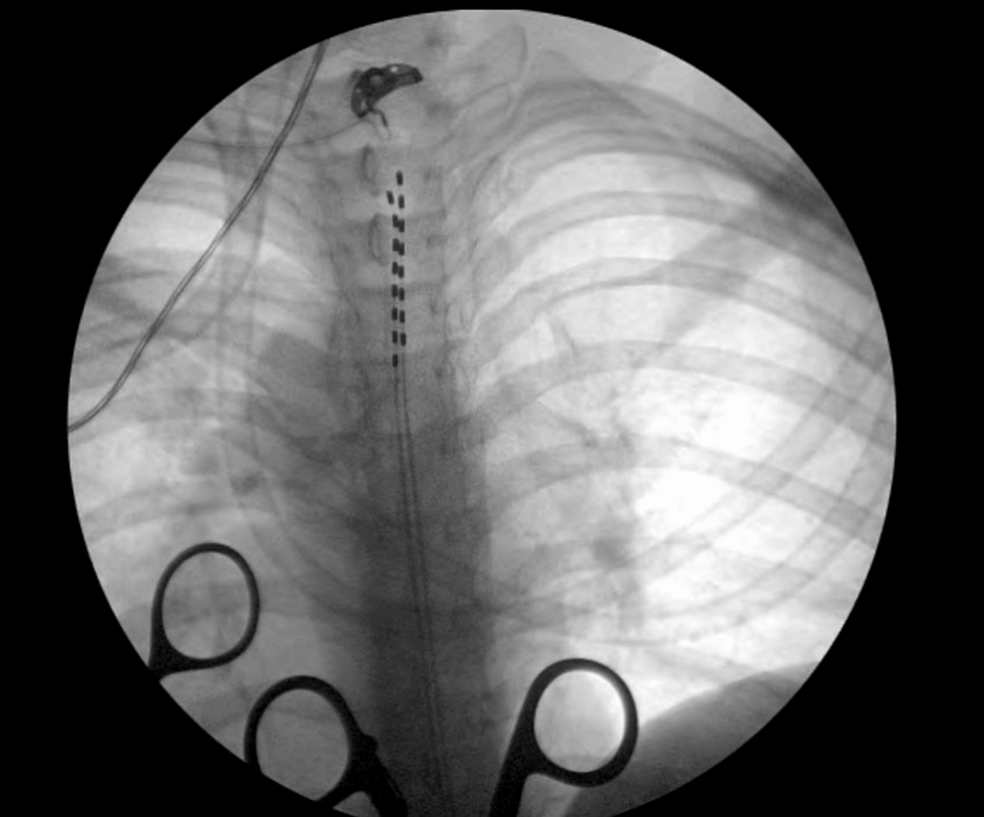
Radiographic evidence of SCS implantation with lead placement SCS: spinal cord stimulator
